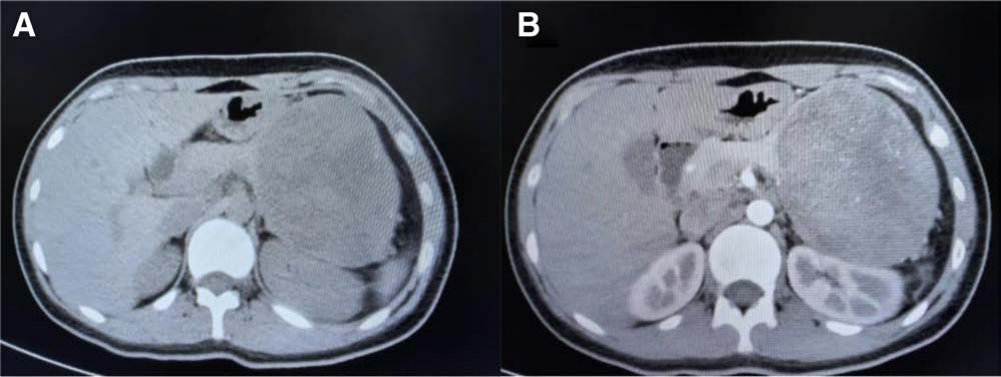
Abdomen CT scan with and without contrast showed a well-defined rounded hypodense non-enhancing parenchymal solid mass lesion in the body and tail of the pancreas, measuring approximately 10.5 cm × 8.2 cm × 10.4 cm. The mass involves the left kidney and compresses it backward and downward. (A) Plain CT scan; (B) contrast-enhanced CT. CT = computed tomography.
